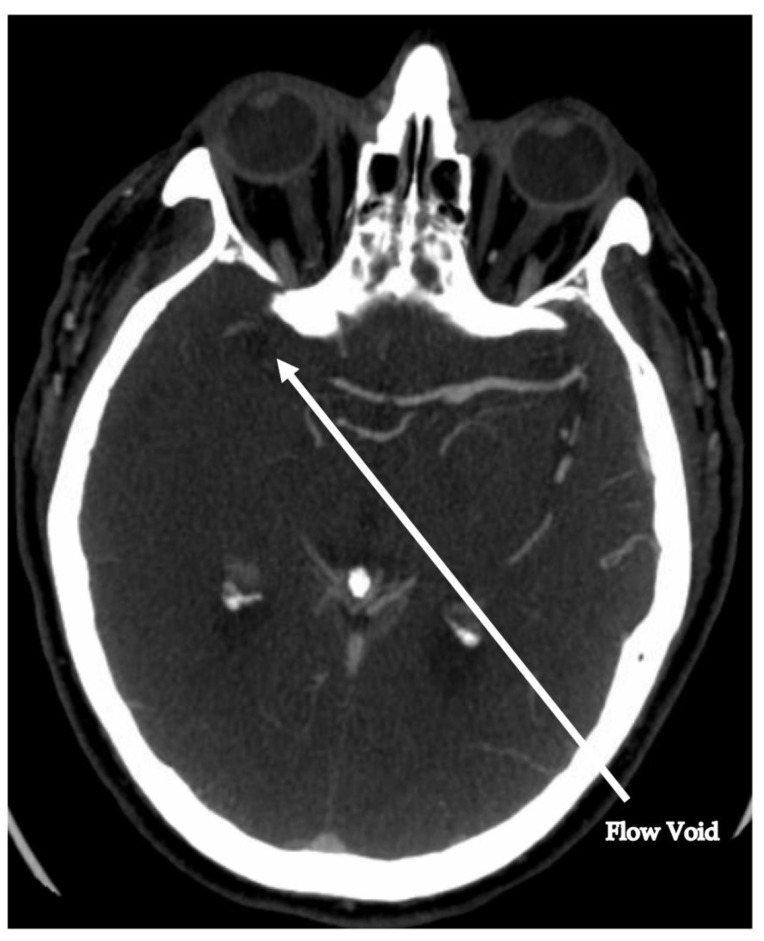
CT angiogram showing flow void in the right internal carotid artery.
